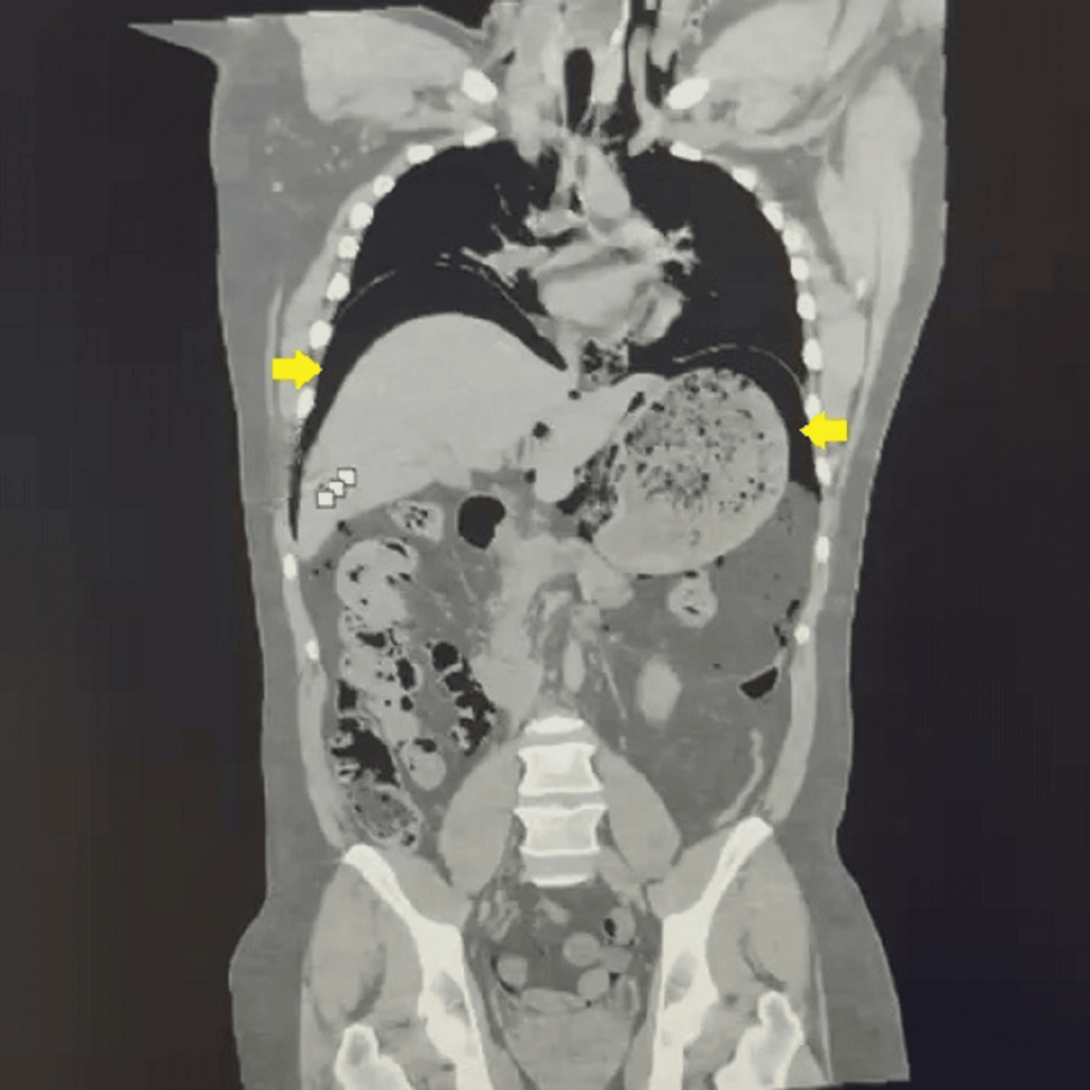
Abdominal CT scan showing pneumoperitoneum (yellow arrows)
